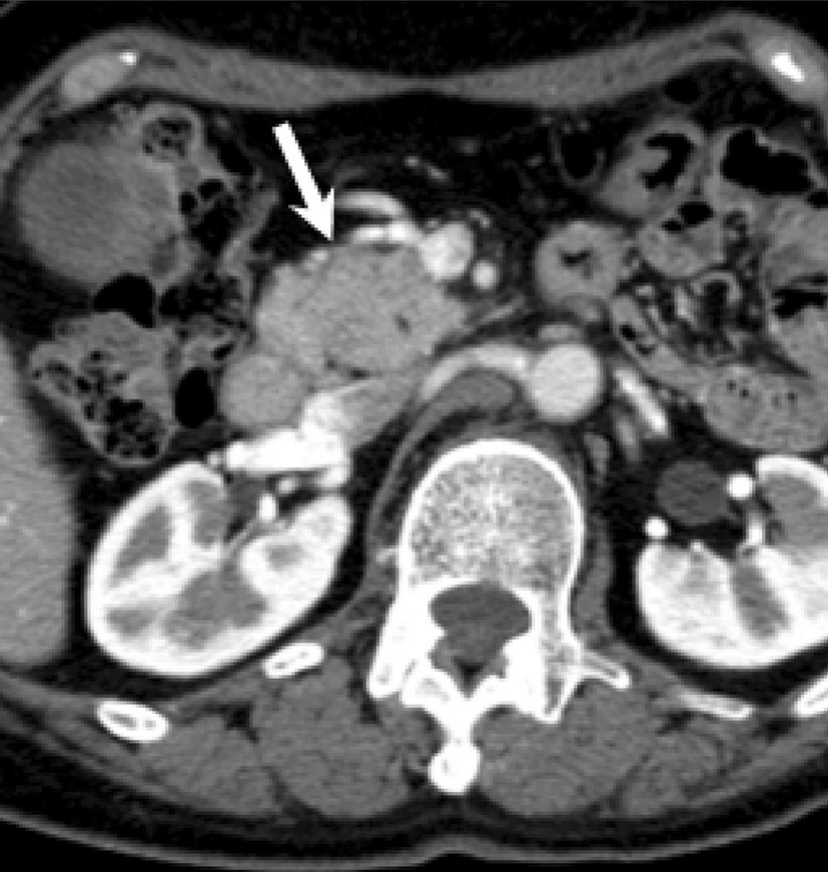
Contrast-enhanced computed tomography (CE-CT) findings at diagnosis of IgG4-related disease. CE-CT revealed a mass in the pancreatic head when she was first referred to our hospital with obstructive jaundice 6 years earlier
